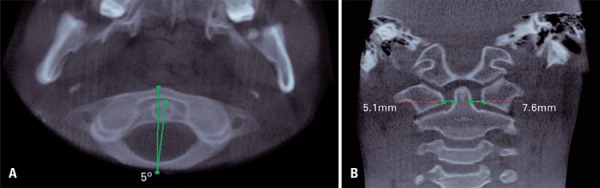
Cone beam computed tomography showing 5º of axial rotation in relation to medium line (A), atlanto-axil space of 5.1 and 7.6mm (B)
